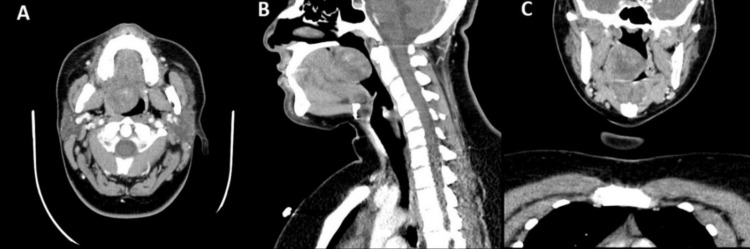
A: Axial, B: Sagital, C: Coronal cuts of the computed tomography (CT) scan with contrast showed a large soft palate mass lesion with heterogeneous enhancement narrowing the airway
